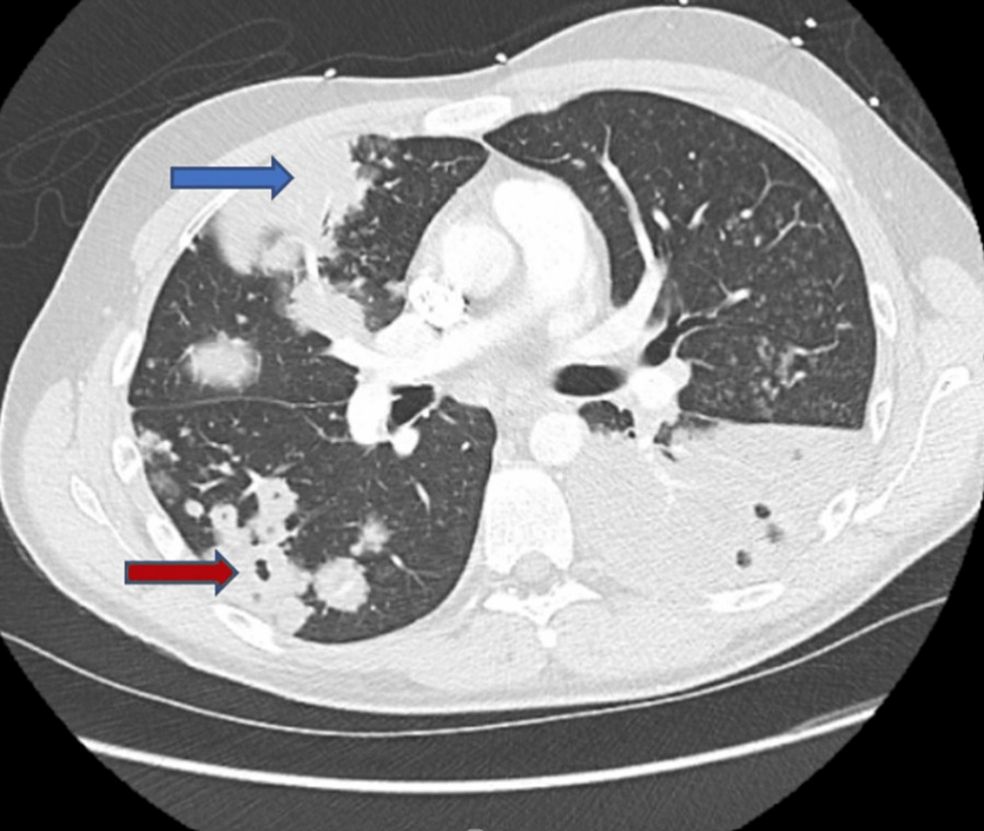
Computed tomography images of extensive multifocal pneumonia with dense right upper lobe and left lower lobe consolidation Multiple areas of breakdown and evolving abscesses within the consolidated left lower lobe (blue arrow). Numerous bilateral pulmonary nodules with cavitating nodules in the left lobe (red arrow).
